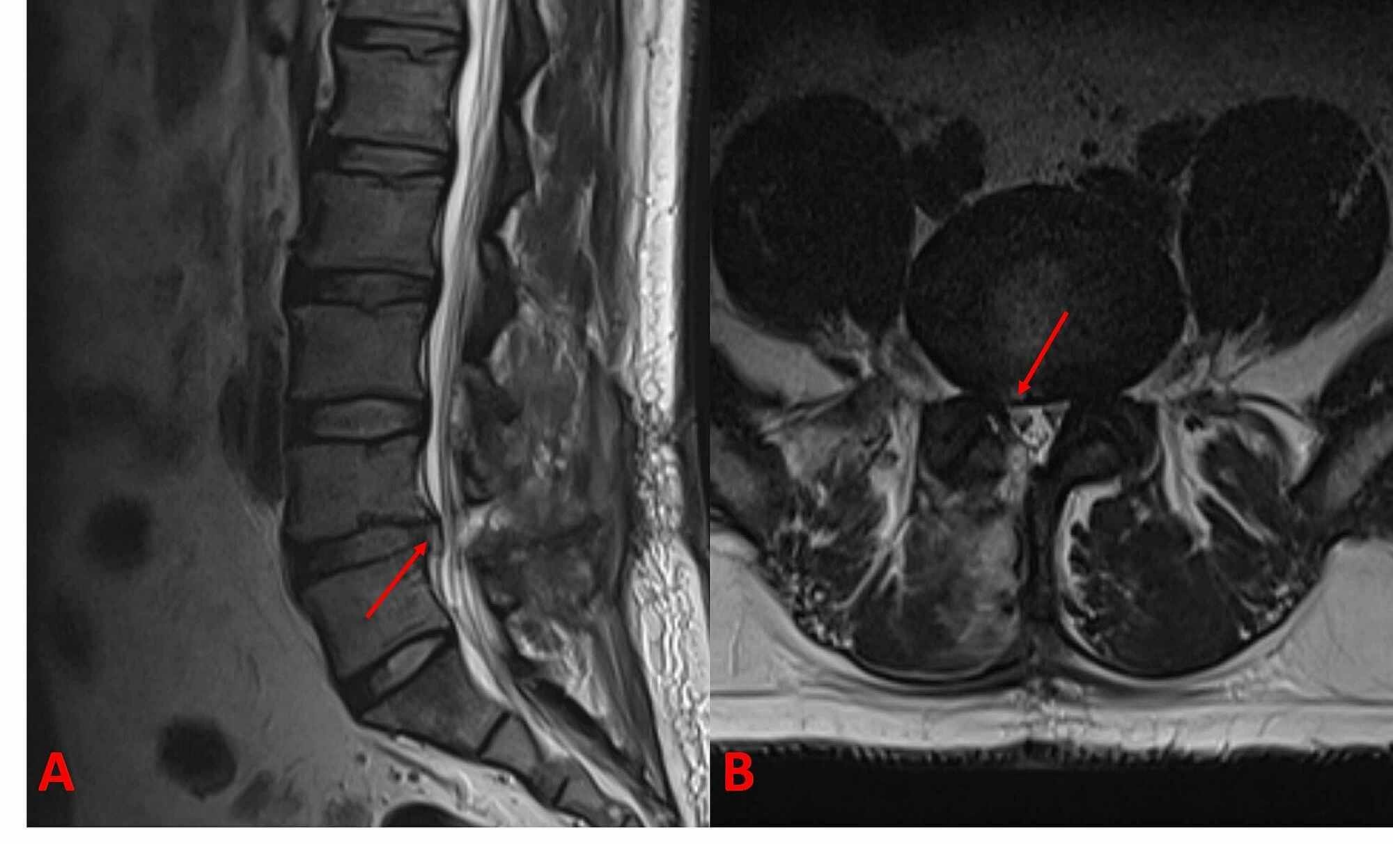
(A) Sagittal and (B) axial MRI sections of a patient with recurrent L4-5 disc herniation. Red arrows show the herniated fragment in each section. MRI: magnetic resonance imaging
